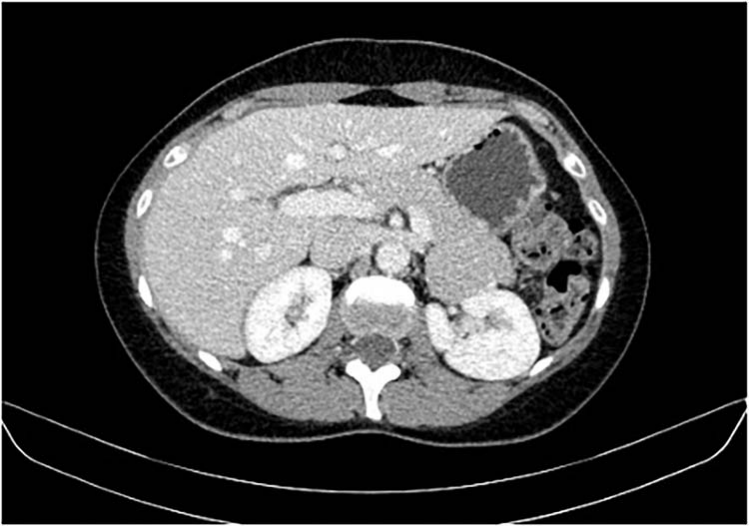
CT scan showing the same mass as shown in Fig. 2 but with washout in the portal venous phase.
